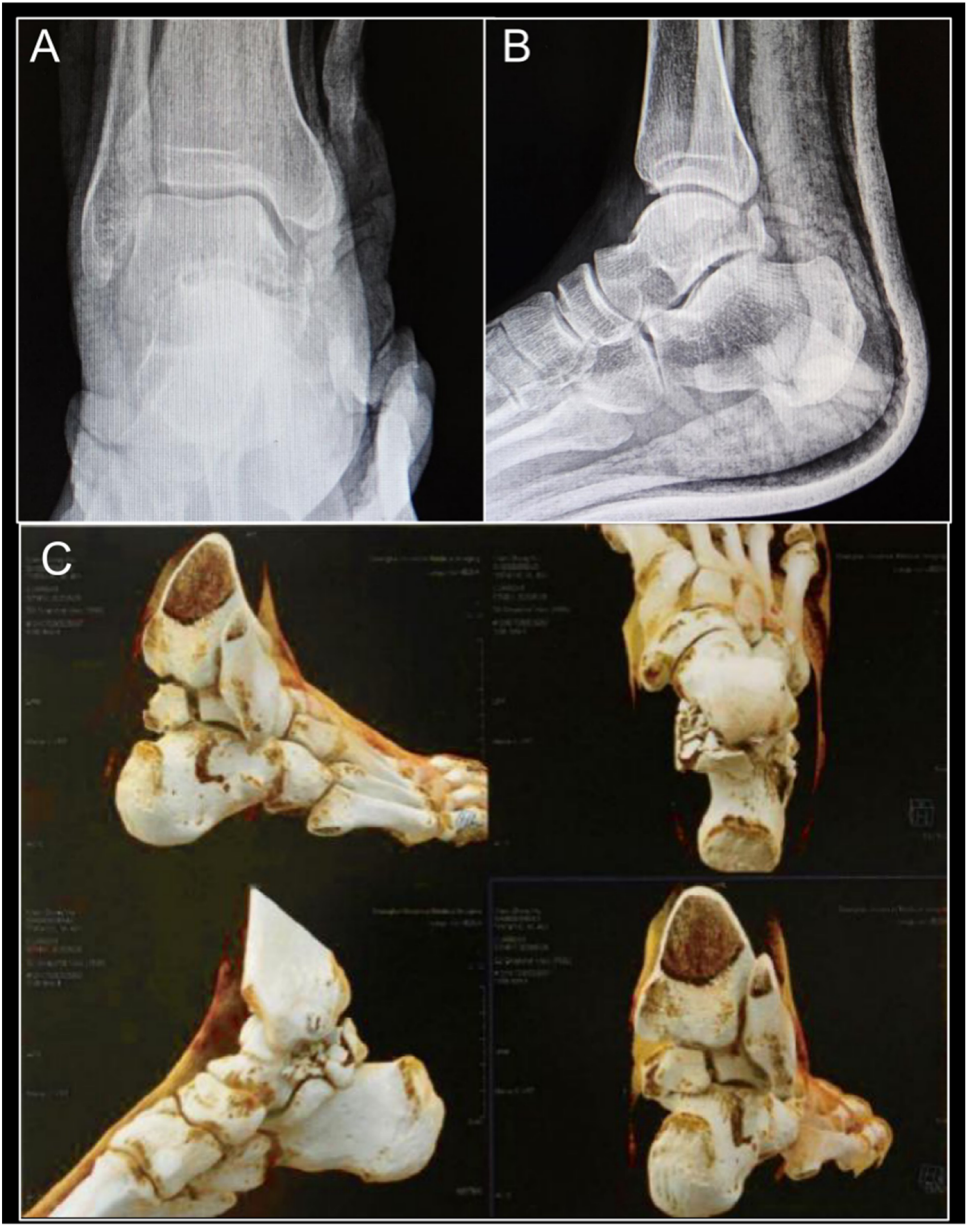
Anteroposterior (A) and lateral (B) x-rays showing a talar fracture; 3D-CT (C) scan showing fractures of the medial and lateral tubercles of the posterior talus and calcaneofibular ligament avulsion fracture.
